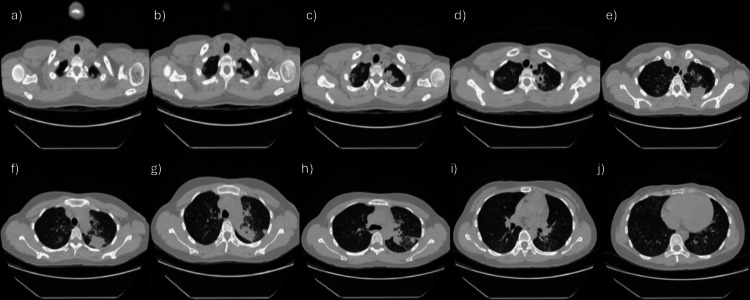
Chest CT without contrast. (a-c) Upper thoracic slices demonstrating cavitary lesions and nodular infiltrates predominantly involving the upper lobes. (d-f) Mid-lung slices demonstrating bilateral tree-in-bud and centrilobular nodularity consistent with endobronchial spread of infection. (g-j) Inferior thoracic slices demonstrating multifocal consolidation and diffuse nodular opacities with right apical pleural thickening.
